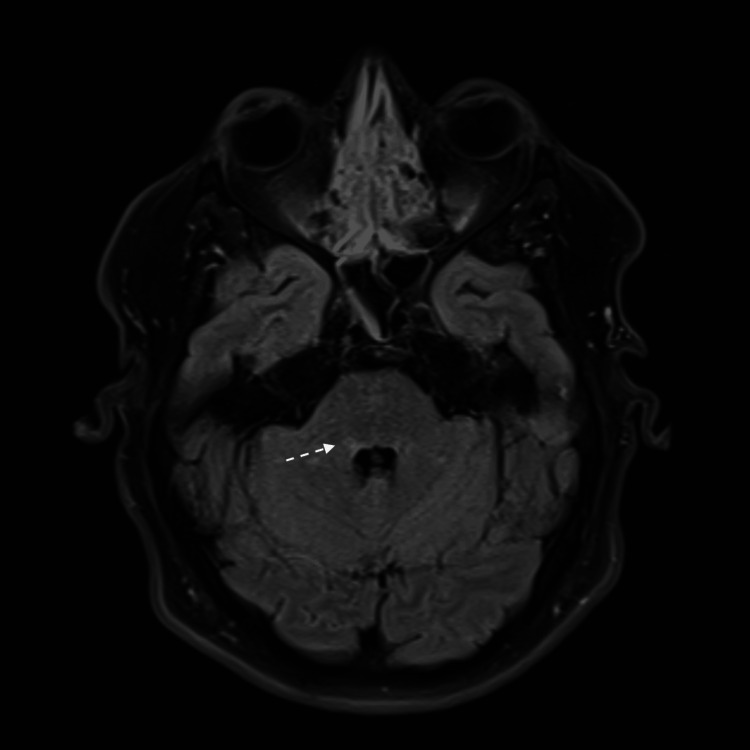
MRI brain FLAIR view showing small left pontine insult.
